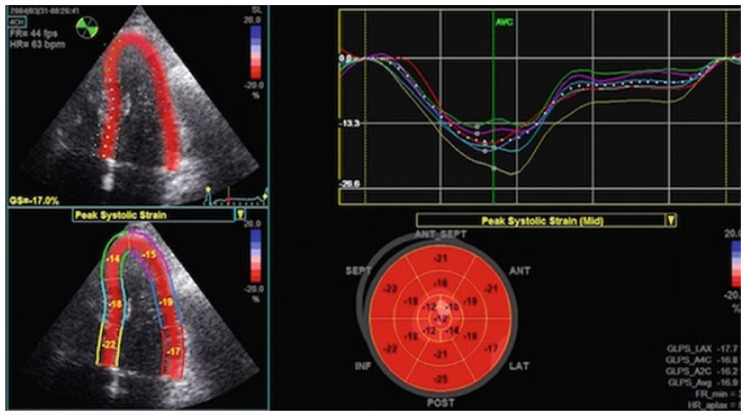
Left: LV 4-chambers view divided into six segments by the STE software https://www.gehealthcare.it/products/ultrasound/vivid/echopac/applications (accessed on 30 November 2023), each providing its own longitudinal strain value. Top: the curves of each segments’ longitudinal strain and the GLS’ curve. Bottom: the “bull’s eye”, the LV divided into seven segments, each with its own longitudinal strain; the GLS of each view and the average GLS is displayed on the right. LV: left ventricle; STE: speckle tracking echocardiography, GLS: global longitudinal strain.
